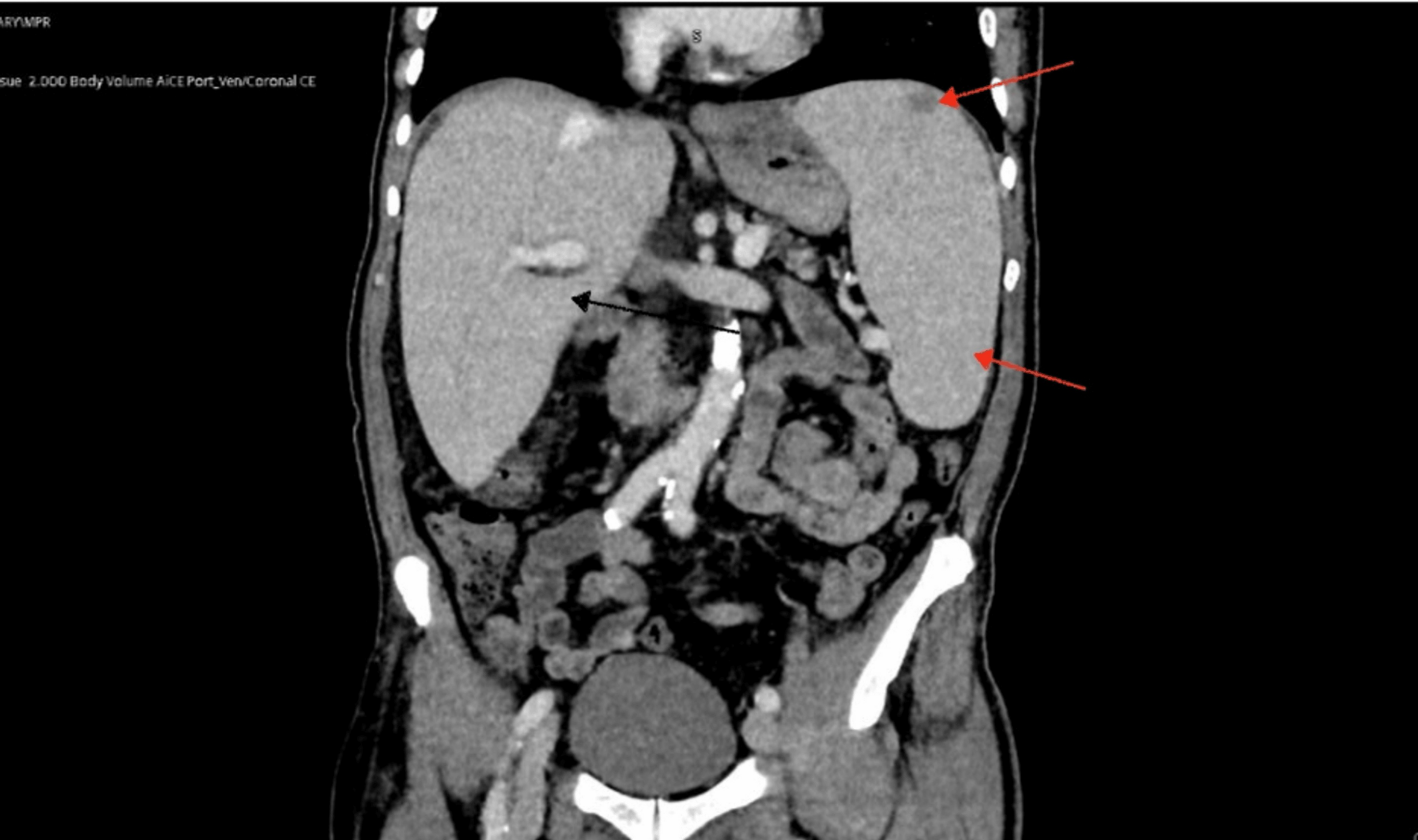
Computed tomogram thorax abdomen and pelvis (CT TAP) showing hepatomegaly (black arrow) splenomegaly (bottom red arrow) and hypodense lesion in the dome of the spleen (top red arrow)
